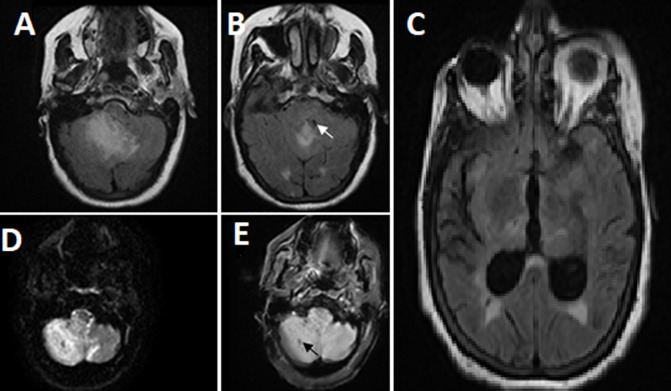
brain MRI revealing a right cerebellar stroke with evidence of hemorrhagic infarction: (A,B,C) FLAIR axial slice demonstrates a hyperintense right cerebellar cortico-subcortical signal with a discrete mass effect on the fourth ventricle, with moderate tri-ventricular hydrocephalus (arrow); D) diffusion imaging in axial section shows a hyperintense signal in the infarcted region in relation to a decrease in apparent diffusion coefficient; E) T2 gradient echo shows a small hypointense focus in the right cerebellum indicating a small hemorrhagic transformation (arrow)
